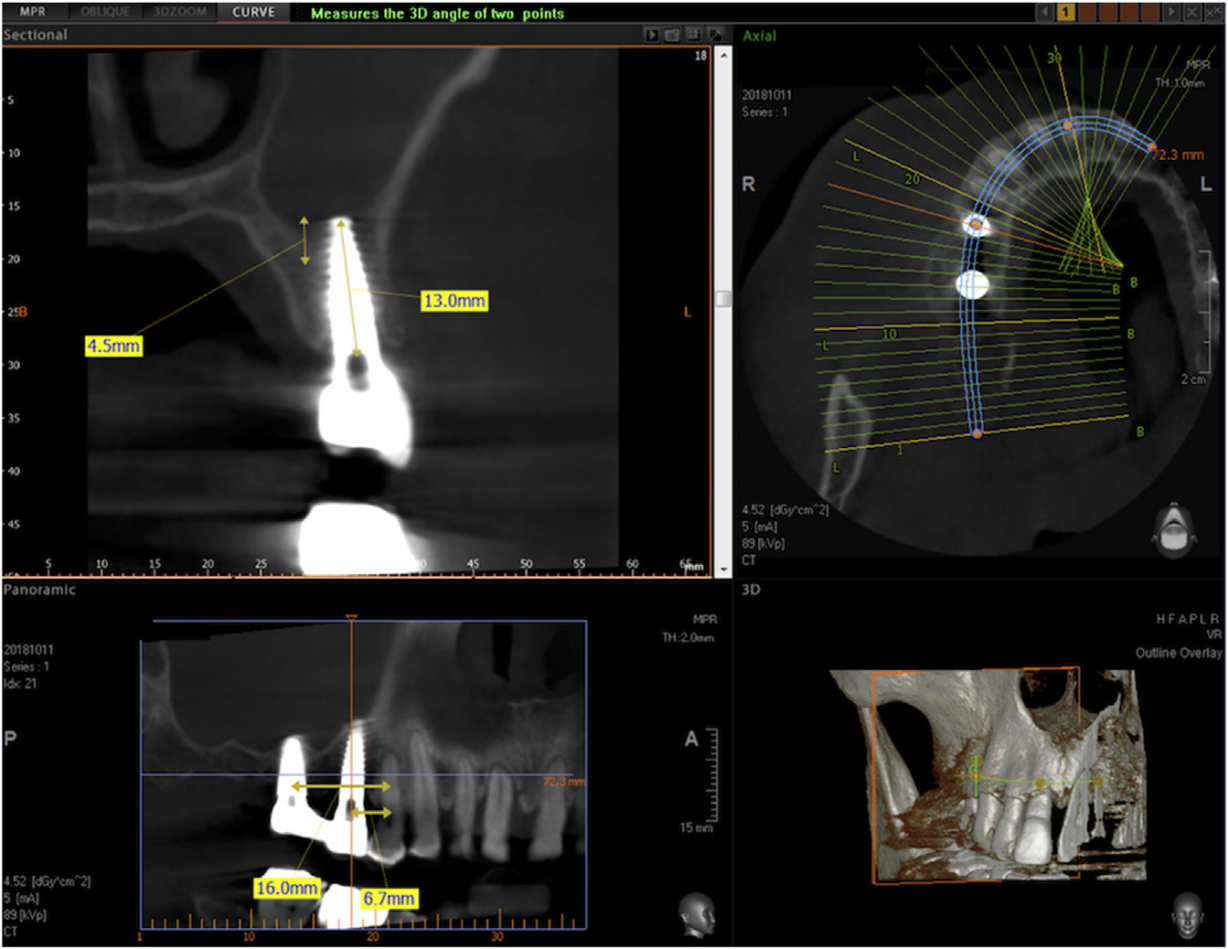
Detail of the CBCT of Figure 12 centered on the implant in the second bicuspid site. The implant is a 3.8 × 15 mm Sweden & Martina UXR. The apex of the implant is covered with newly formed bone. The vertical bone augmentation is about 4.5 mm.
